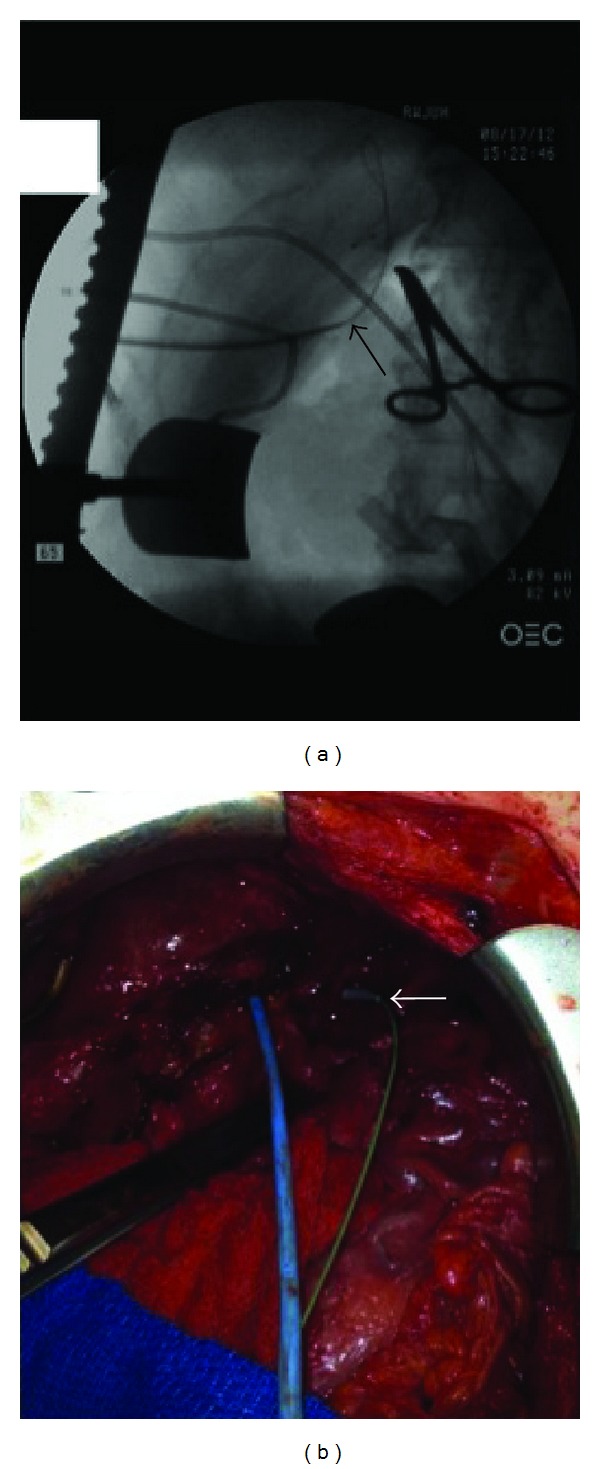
Intraoperative radiographically guided tunneling of biliary catheters. (a) Intraoperative radiograph showing interventional radiologist tunneling 16 gauge Colapinto needle through the previously placed posterior sectoral sheath (Cook Medical Inc, Bloomington, In). A guidewire traversing regenerated liver into the peritoneal cavity is demonstrated (arrow). (b) Operative field view with a catheter in the anterior ductal system inserted retrograde from the bile duct and out the liver surface and abdominal wall (arrow) and the posterior catheter inserted from an outside-in direction.
